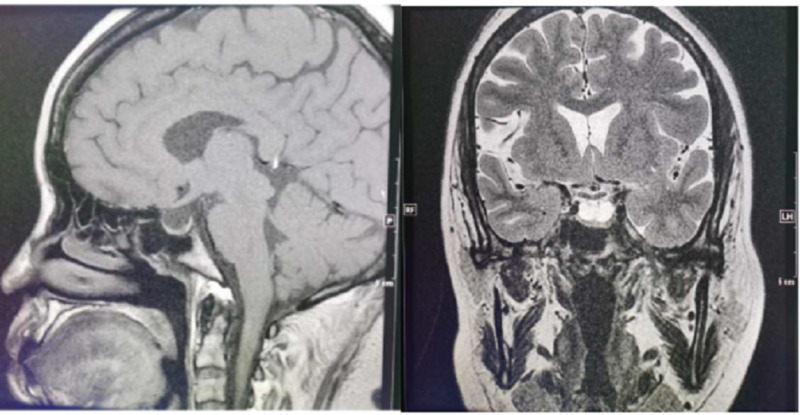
Pituitary magnetic resonance imaging (MRI)
